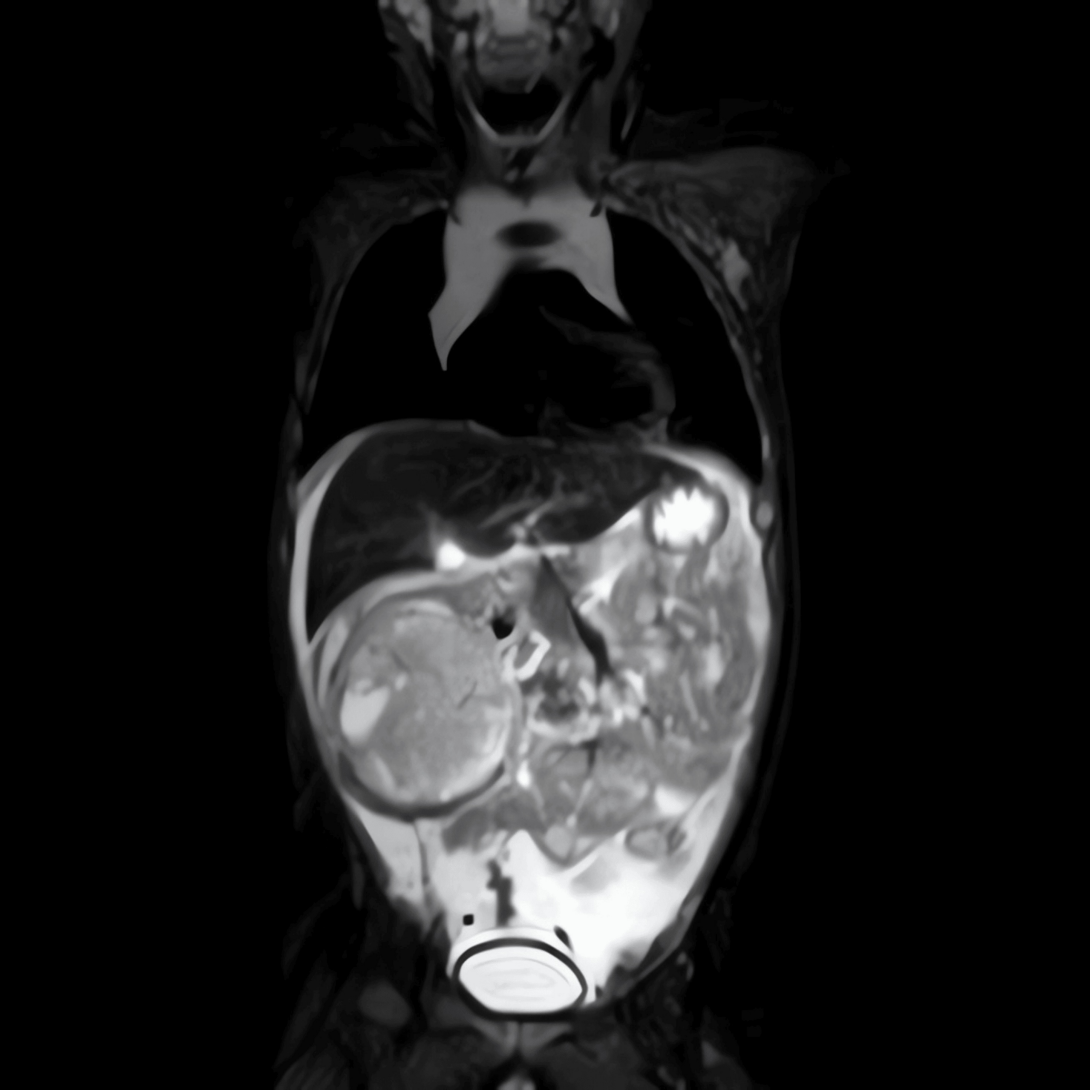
Coronal MRI of the abdomen with further characterization of the heterogeneous intrarenal mass and associated ascites
